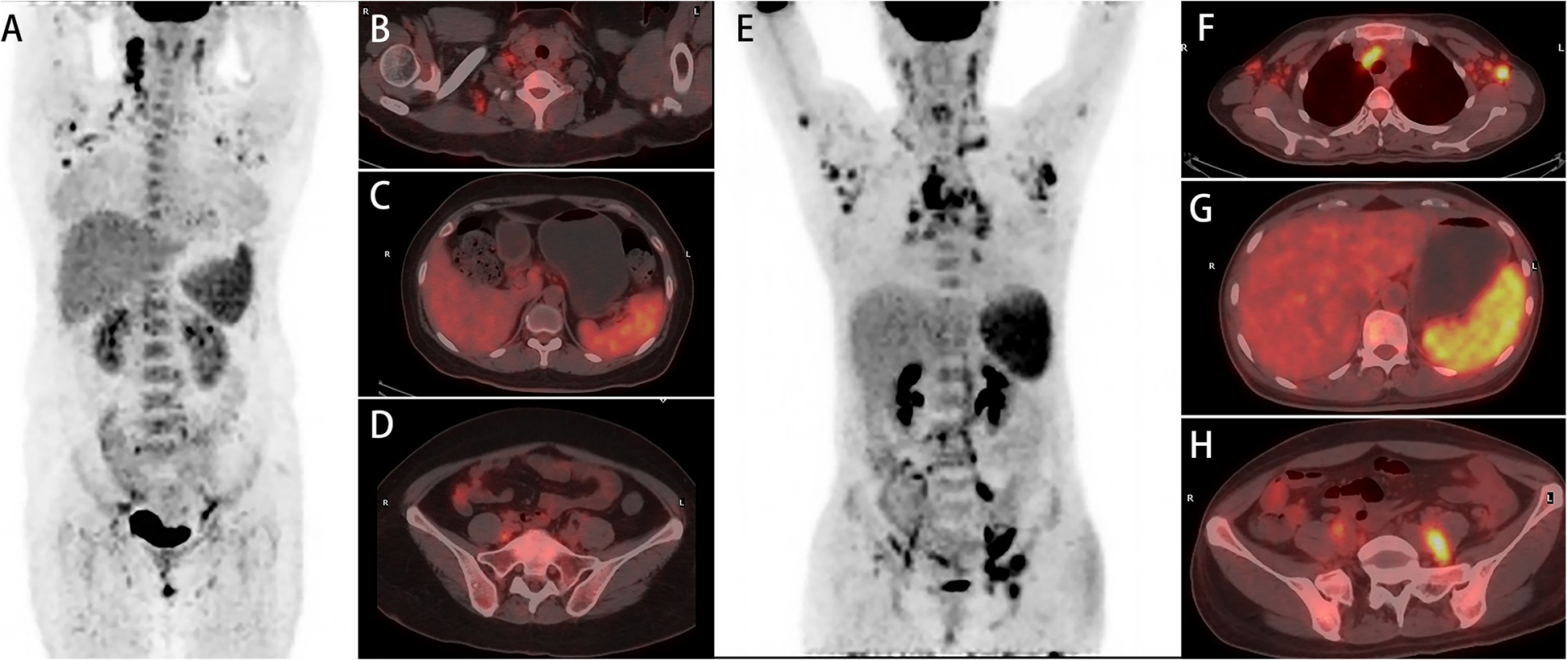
Whole-body PET image (a) and axial hybrid PET/CT images of the axillary LNs (b), liver and spleen (c), and pelvis bone (d) from a 45-year-old female patient with fever, skin rash, and arthralgia showed increased ^18^F-FDG uptake by the right axillary LNs (SUV_max_, 6.7), spleen (SUV_max_, 4.7), liver (SUV_max_, 3.3), and pelvic bone marrow (SUV_max_, 4.3). The TLG_total_ of LNs was 199,697.4, and MLV_total_ of LNs was 53.88. The patient did not progress to MAS after glucocorticoids and methotrexate treatment. Another 42-year-old female patient with fever, skin rash, and lymphadenopathy showed increased ^18^F-FDG uptake by the left axillary LNs (SUV_max_, 9.8), mediastinum LNs (SUV_max_, 11.4), spleen (SUV_max_, 7.5), liver (SUV_max_, 4.7), and pelvic bone marrow (SUV_max_, 5.1) on whole-body PET image (e) and axial hybrid PET/CT images of the axillary and mediastinum LNs (f), liver and spleen (g), and pelvis bone (h). The TLG_total_ of LNs was 1,144,469.8, and MLV_total_ of LNs was 244.75. The patient progressed to MAS after glucocorticoid treatment. Lately, she received intravenous immunoglobulins and achieved remission. LNs, lymph nodes; SUV, standardized uptake value; TLG, total lesion glycolysis; MLV, metabolic lesion volume; MAS, macrophage activation syndrome
